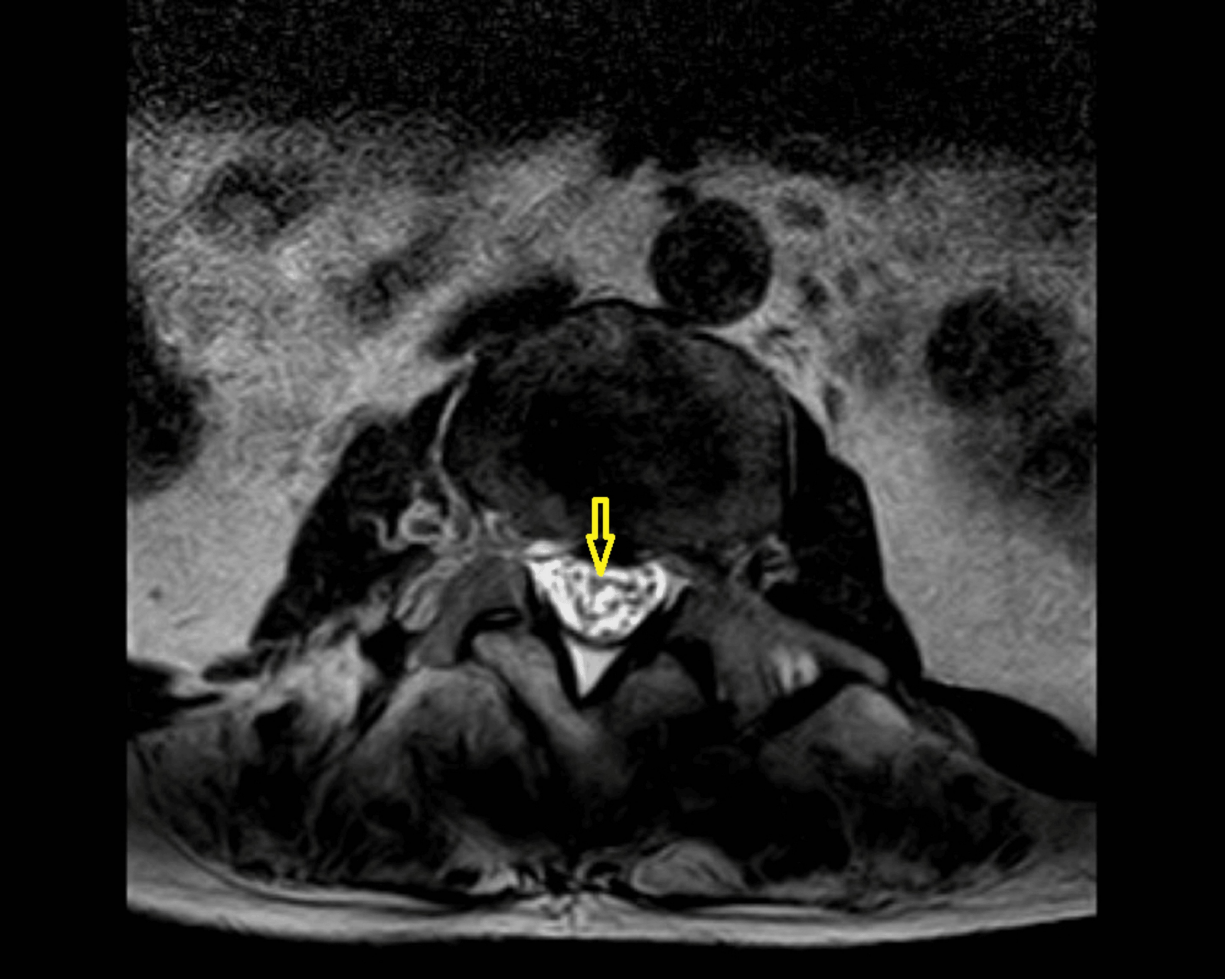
Axial T2-weighted image show displacement of the nerve roots of the cauda equina in the initial images. Abnormal thickening and clumping of the cauda equina with intrathecal hypointense signal abnormality seen at distal lumbar, consistent with sequelae of arachnoiditis. (Image published under Creative Commons License; image credits: case courtesy of Noriza Zainol Abidin, Radiopaedia.org, rID: 51394)
